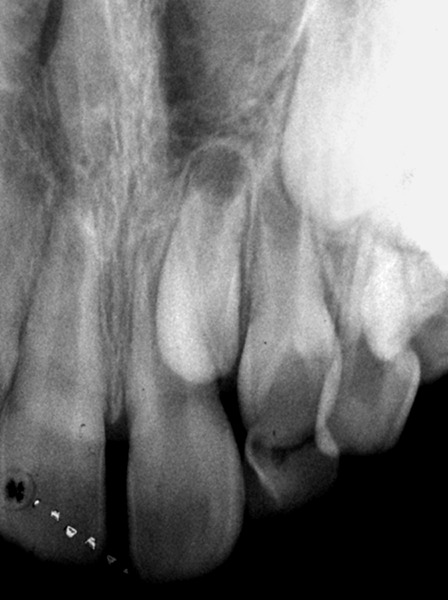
Periapical radiograph showing DE on 62 and an unerupted supernumerary tooth
